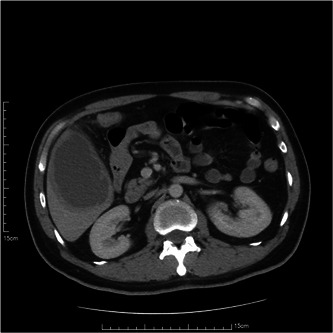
The delayed phases of contrast‐enhanced computed tomography show a low‐density lesion in the right lobe of the liver, measuring approximately 9.3 × 6.1 cm, with thickening of the cyst wall (white arrow).
